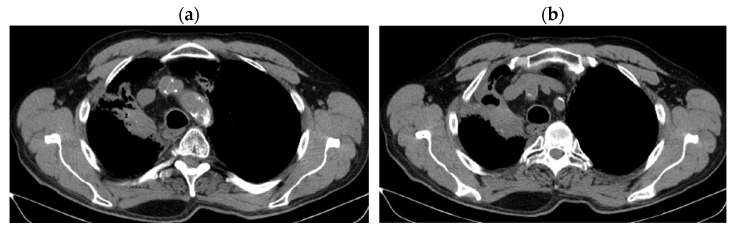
(a,b) CT reevaluation from 23/09/2022 vs. 06/2022.
